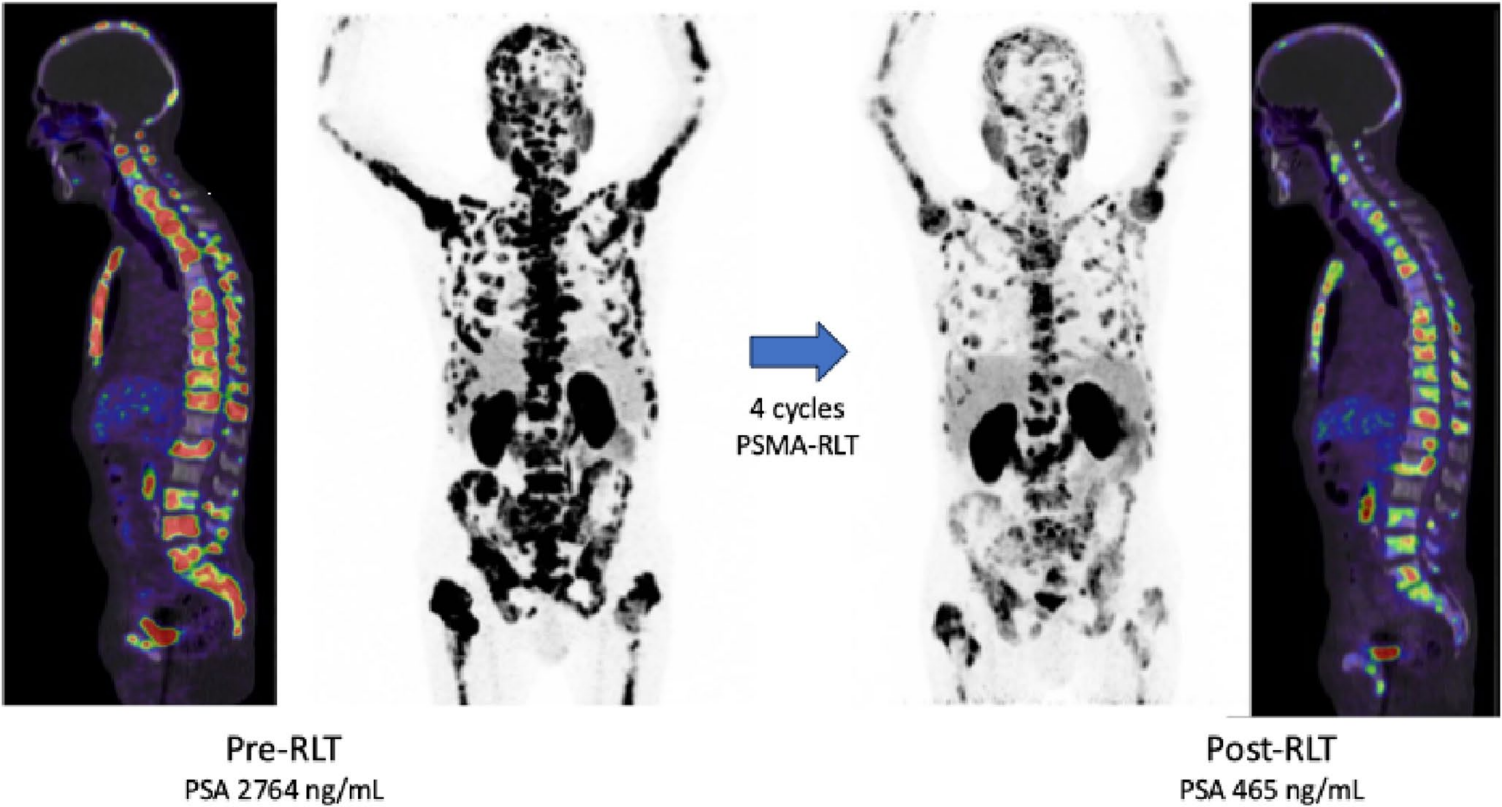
Exemplary maximum intensity projection (MIP) images showing pre-treatment and post-treatment [^68^Ga]Ga-PSMA-PET/CT scans from one patient (76 years old) treated with four cycles of [^177^Lu]Lu-PSMA-617 RLT
